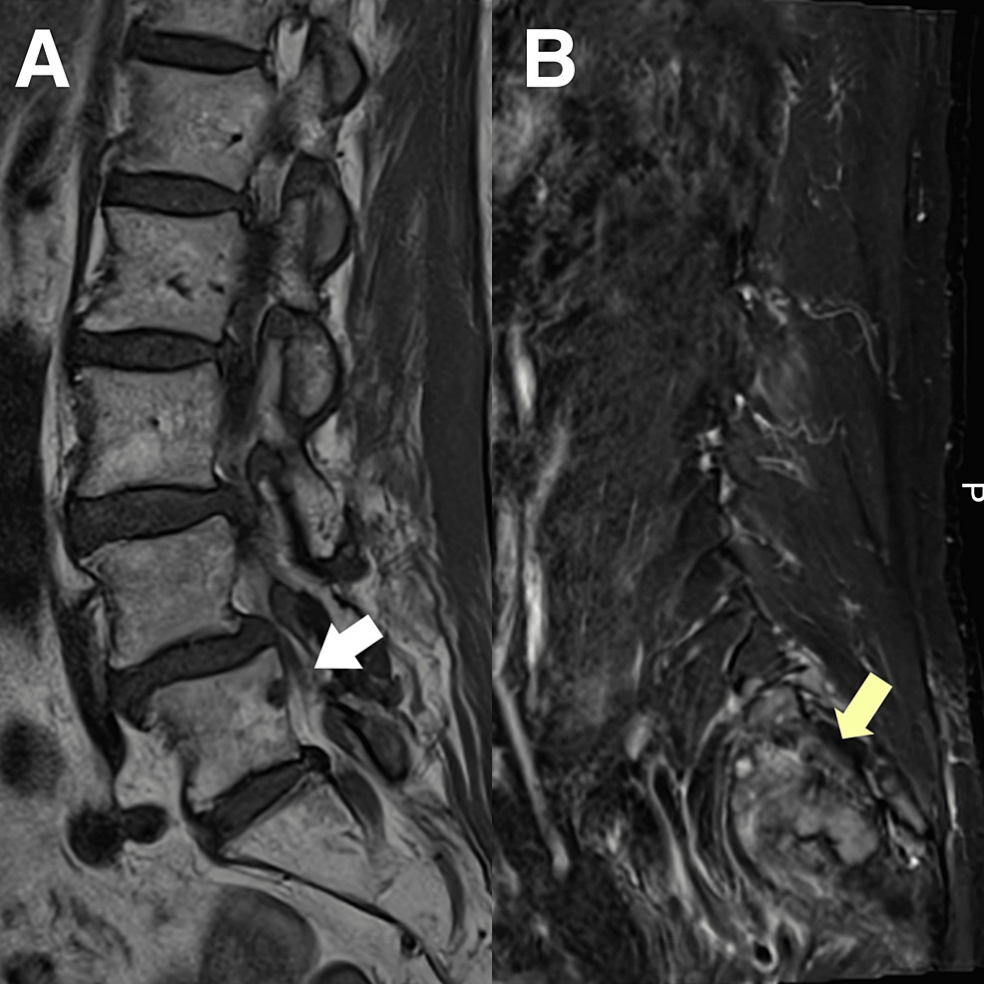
Magnetic resonance imaging (MRI) of the lumbar and sacral spine A) T1W hypointense bone lesions are seen at  L5 vertebral body (white arrow). Grade l L4/5 anterolisthesis. Mild-to-moderate lumbar spondylosis with L2/3, L4/5, and L5/S1 disc height loss. B) Several ill-defined lesions are located at the sacral region (yellow arrow). Collectively, these features are indicative of a diagnosis of strongly suspected bone metastases.
